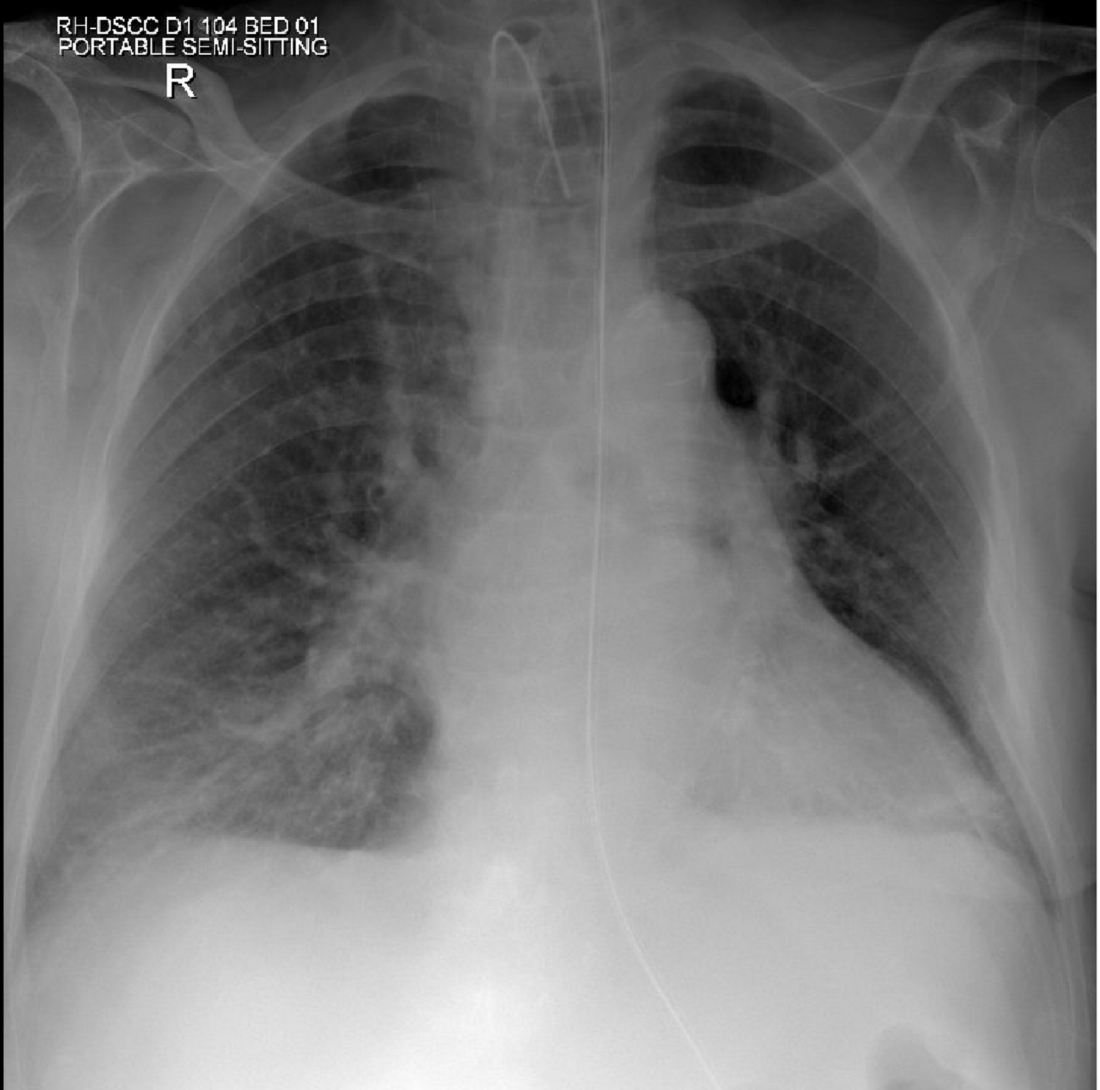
Chest X-ray showing nasogastric tube and tracheostomy tube with no clear evidence of foreign body (radiolucent)
